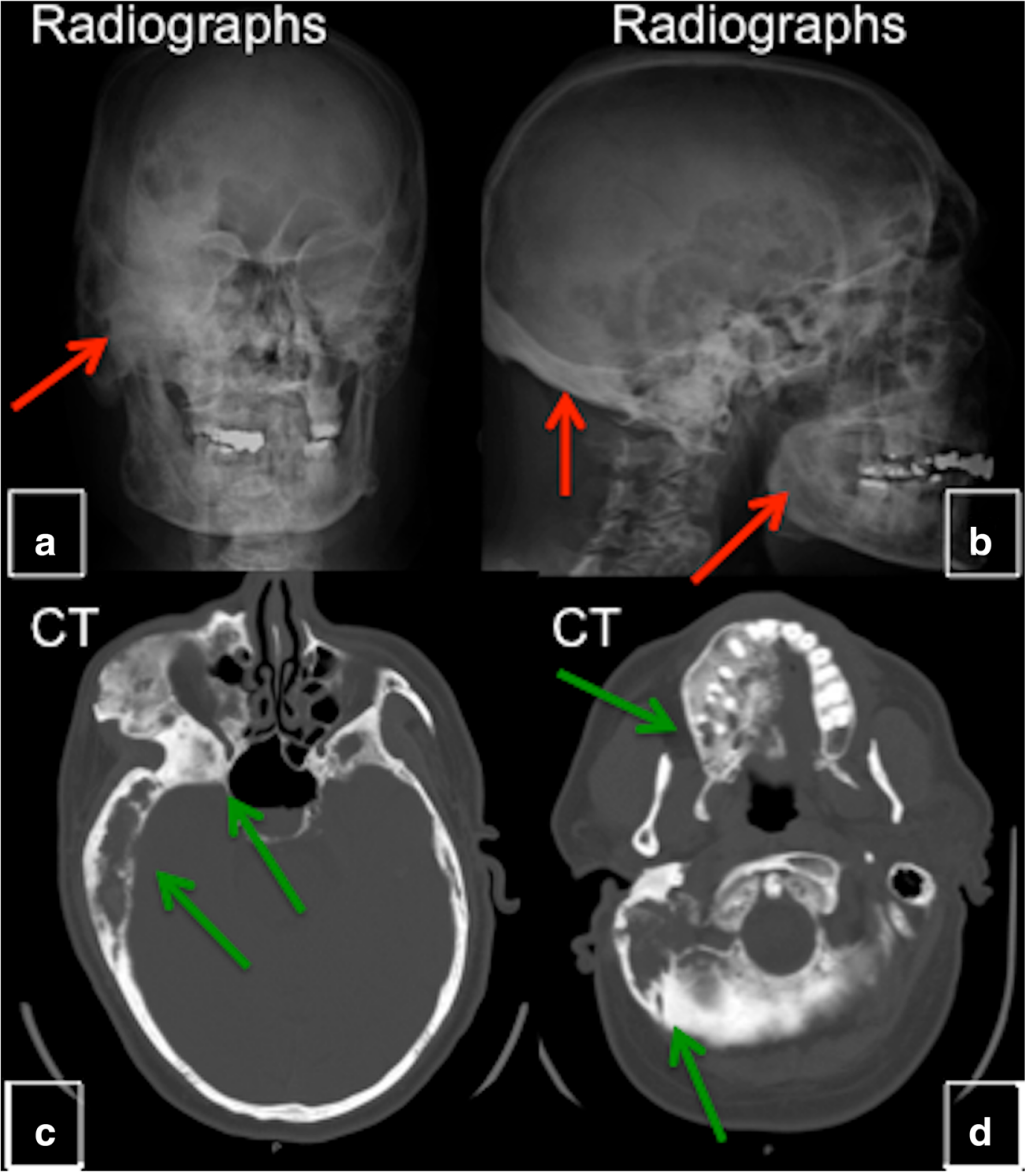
CT in fibrous dysplasia (FD). CT imaging is the modality of choice and superior to radiographs in delineating morphological changes in bone. Radiographs are not recommended for diagnostic purposes or for the characterisation of craniofacial lesions. a, b Radiographs of the head show evidence of FD (*red arrow*). c, d CT of the head on the same patient delineates lesions and demonstrates relationship between lesions and neuronal, vascular and soft tissue structures (*green arrow*)
